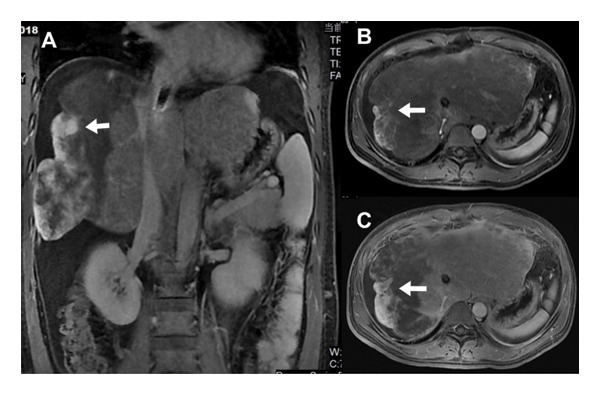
MRI imaging of Case 1: (A) coronal‐enhanced scan in the delayed phase reveals inhomogeneous enhancement of intrahepatic masses, accompanied by perihepatic ascites. (B) Axial T1WI arterial phase scan displays diffusely reduced enhancement of intrahepatic mass shadows. (C) Axial delayed phase scan exhibits contrast agent filling from the margin to the center of the mass in the right lobe of the liver).
